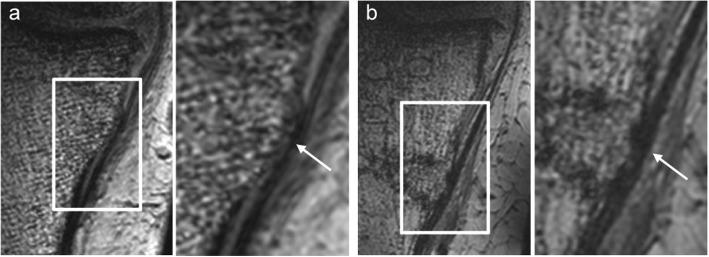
a Preoperative T2-weighted coronal plane MRI showing the sMCL. b After removal of a locking plate, the sMCL (indicated by white arrows) shows iso-intensity and is thicker than on the preoperative image
